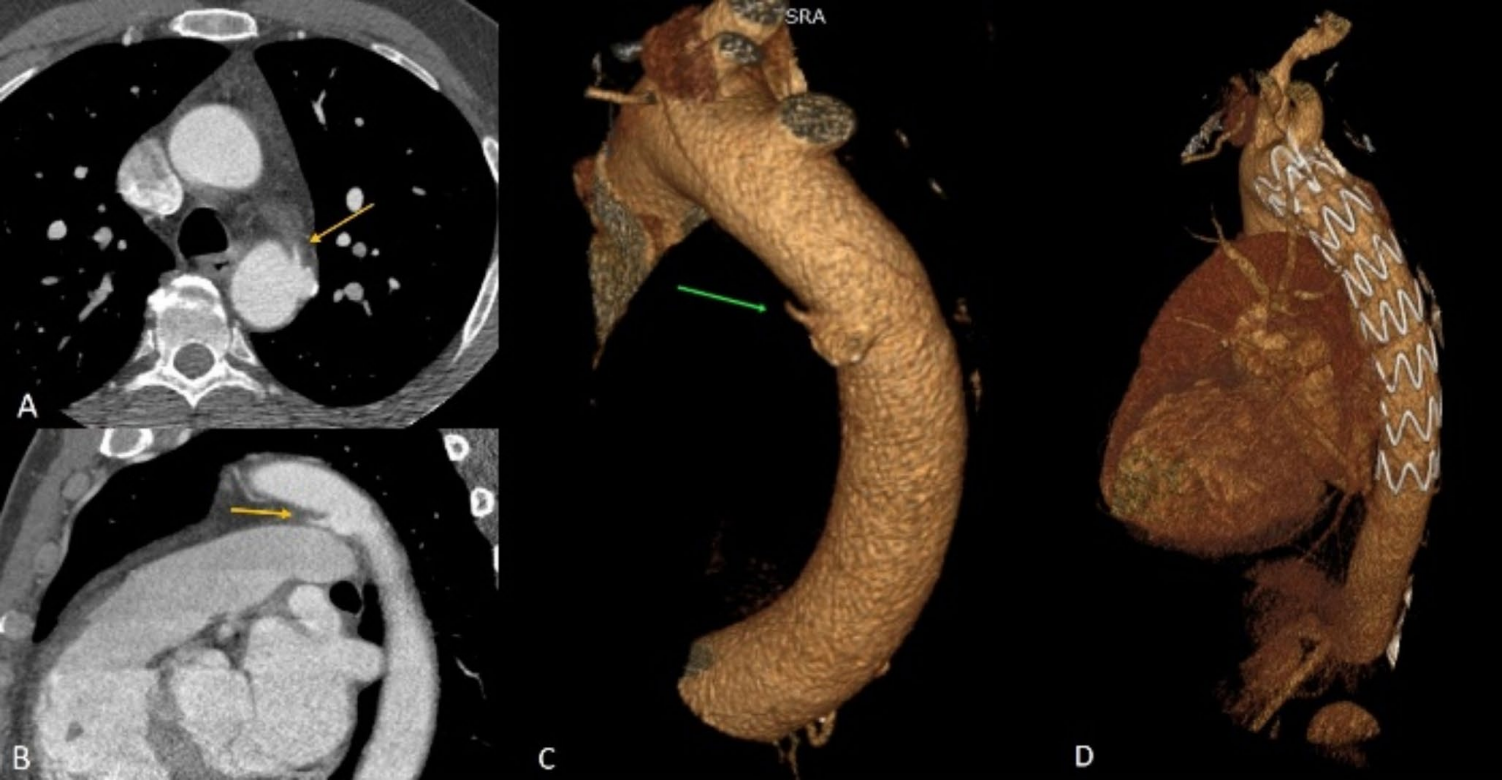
Penetrating aortic ulcer. Axial (A) and sagittal (B) computed tomography angiography (CTA) images of a thoracic penetrating aortic ulceration at the distal aortic arch / most proximal descending aorta (yellow arrow). Volume rendered images of the thoracic aorta with the ulceration pre-intervention (C) and post repair with TEVAR (D). Post intervention images showing no evidence of residual penetrating aortic ulceration
